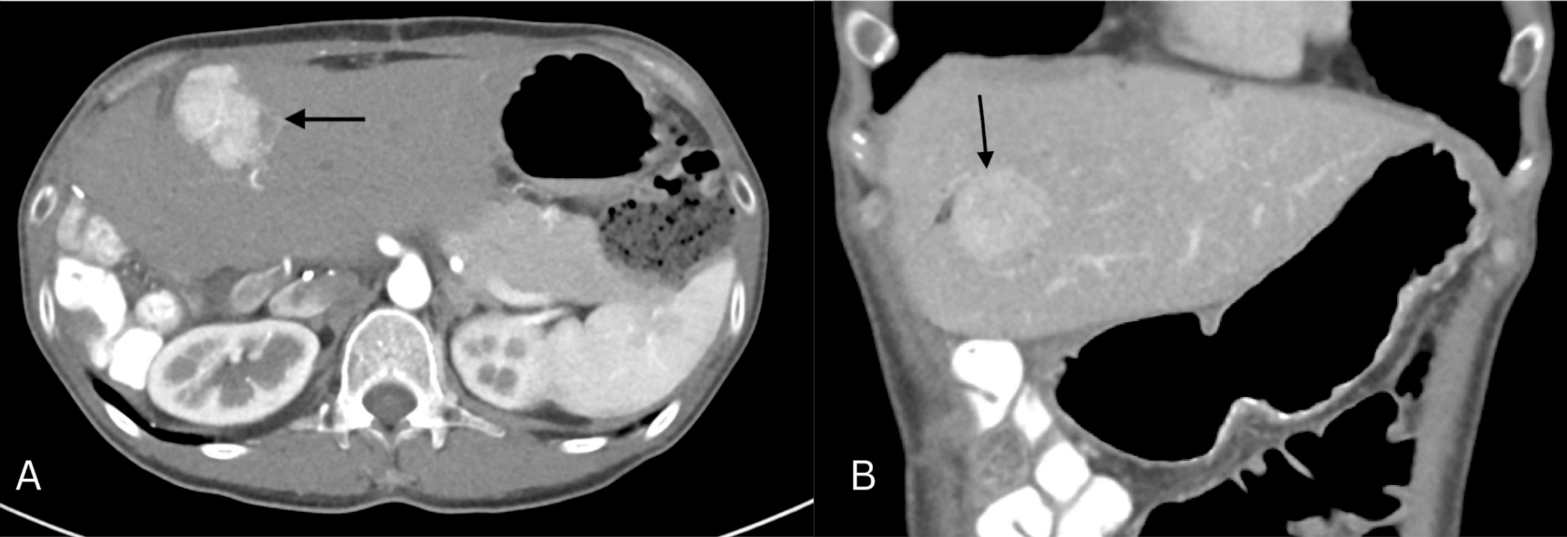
Contrast-enhanced CT of the liver, black arrows pointing at FNH. (A) Arterial phase: hyperdense lesion compared to surrounding parenchyma. (B) Venous phase: isoattenuation CT: computed tomography, FNH: focal nodular hyperplasia
